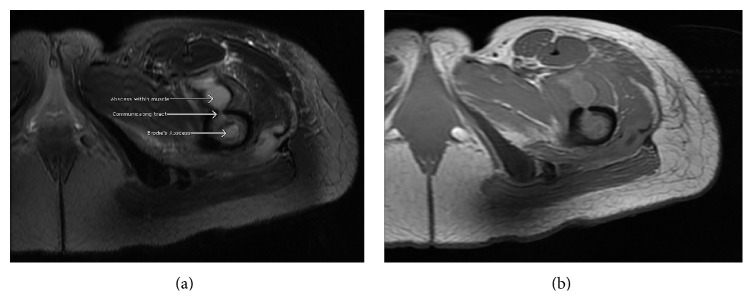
Axial views of MRI showing the Brodie's abscess in the left proximal femur, with a break in the cortex and a tract communicating with the abscess and overlying muscle. (a) T2-weighted image; (b) T1-weighted image.
